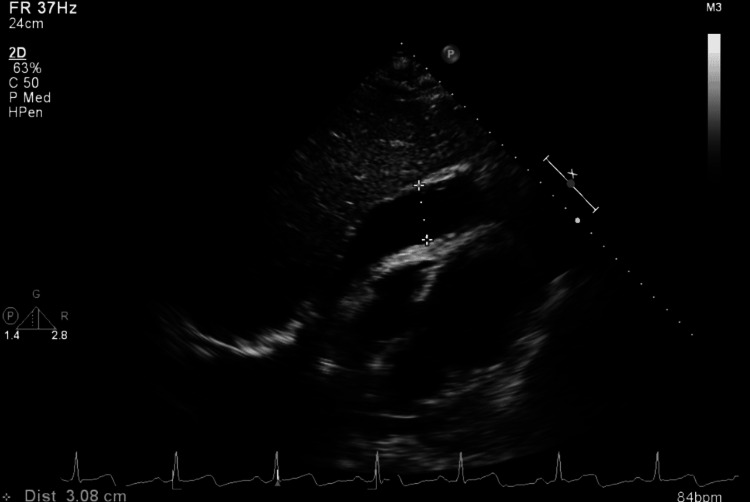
Transthoracic echocardiogram in subcostal four-chamber view demonstrating large loculated pericardial effusion.
